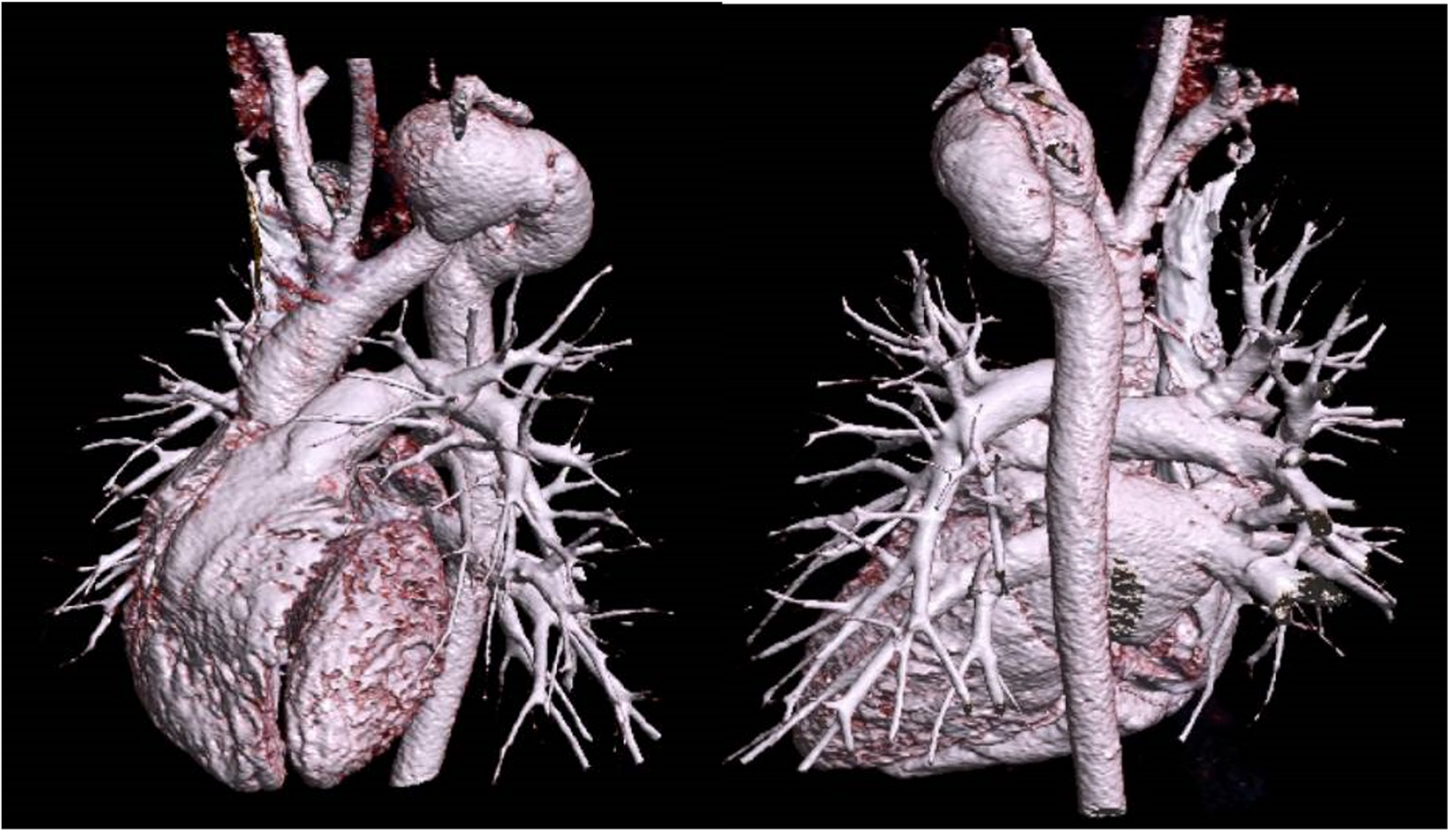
CT scan 3D reconstruction of the author's institutional cervical aortic arch case. 3D reconstructed CT scan showing the left-sided cervical aortic arch anatomy of the institutional patient included in the analysis.
